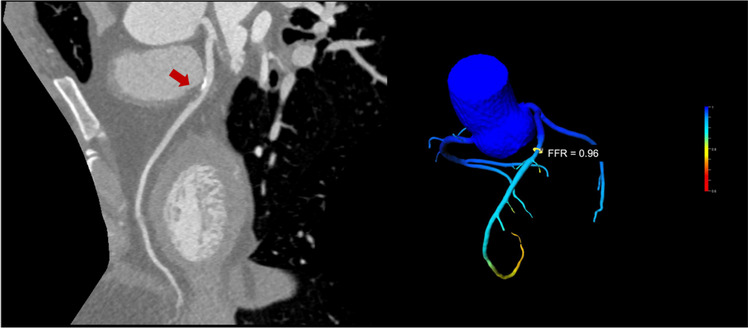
Functional testing using CT-FFR. The image on the left shows an intermediate stenosis of the left anterior descending artery by a mixed plaque. Using CT-FFR as shown on the right, it was possible to exclude haemodynamic relevance of the stenosis
